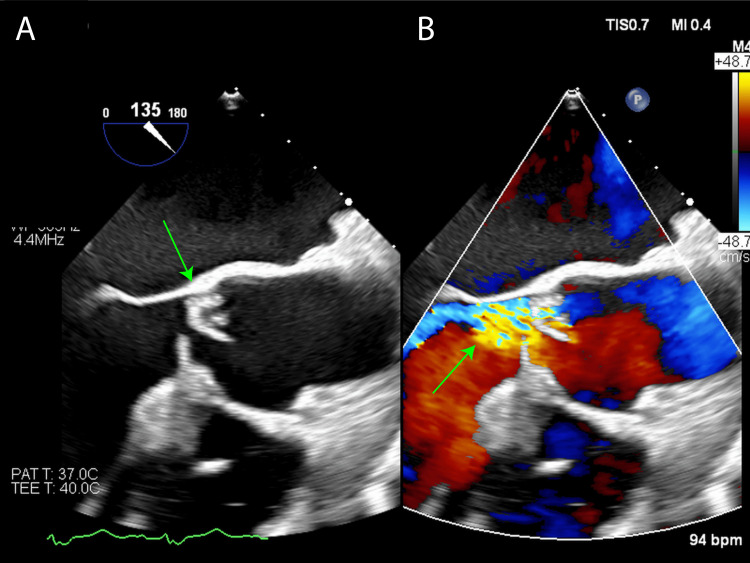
Intraoperative transesophageal echocardiography images (A) Large vegetation attached to the right coronary cusp of the aortic valve (green arrow). (B) Perforation observed on the right coronary cusp of the aortic valve, with a prominent jet of aortic regurgitation (green arrow).
